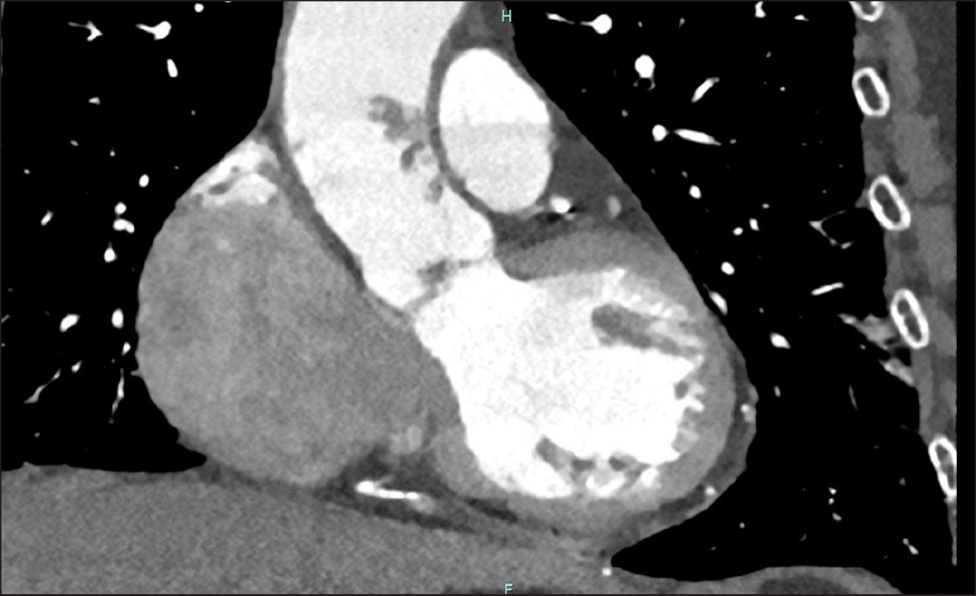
Longitudinal axial computed tomographic angiography image revealing a pedunculated aortic mass in the ascending aorta.
